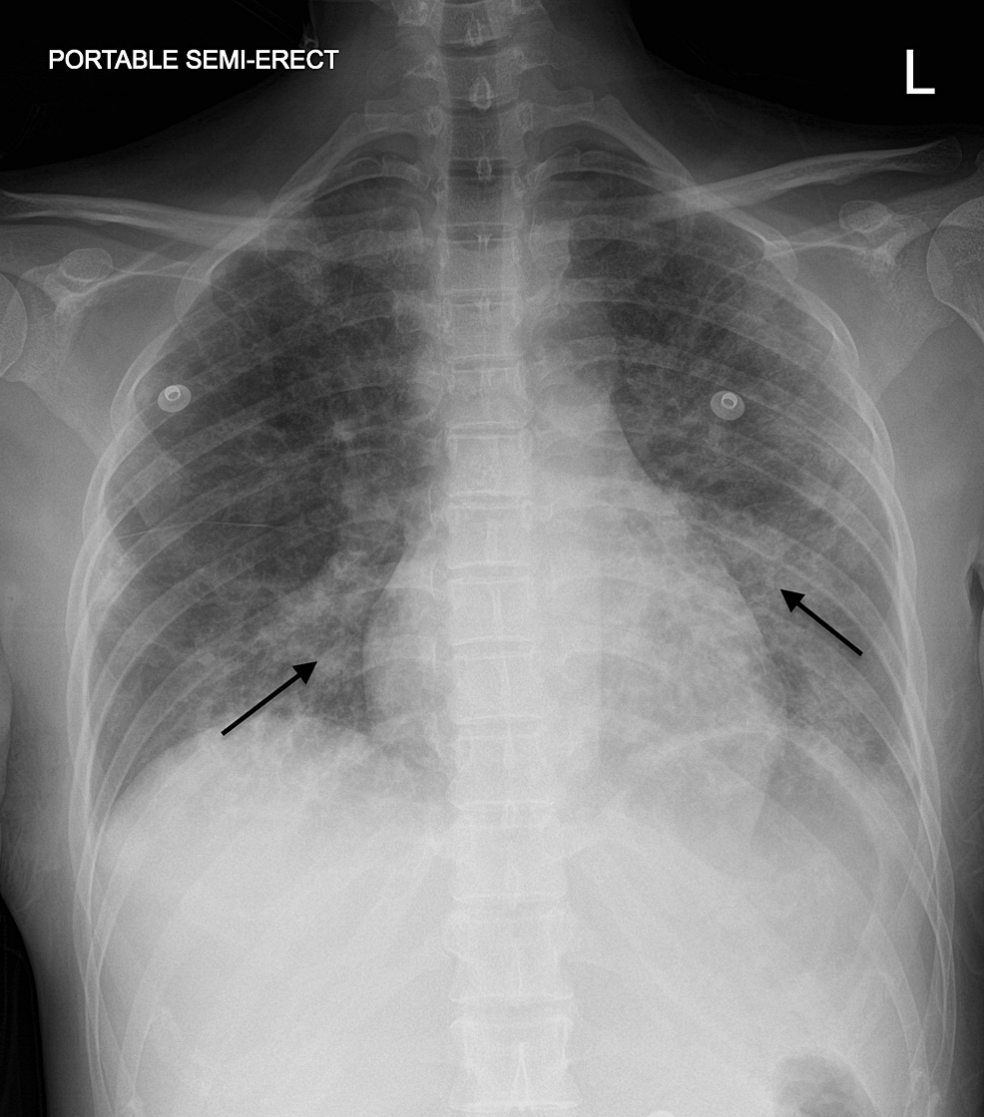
The chest X-ray revealed bilateral interstitial infiltrates (see arrows)
